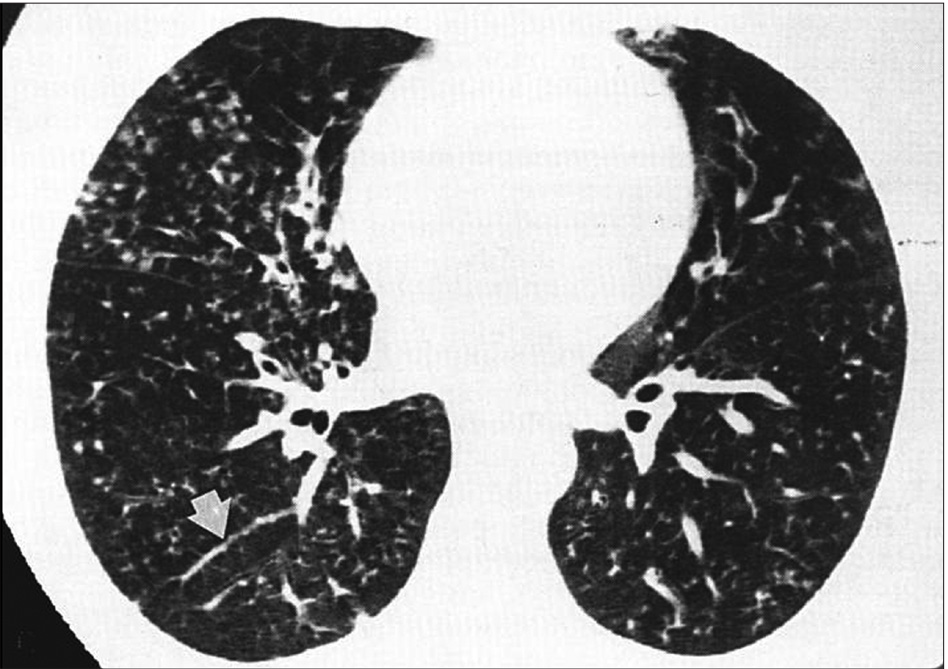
Lymphocytic interstitial pneumonitis in a human immunodeficiency virus patient. High-resolution computed tomography shows smooth and nodular thickening of bronchovascular bundles, centrilobular and subpleural nodularity, ground-glass opacification and interlobular septal thickening. Cysts and mediastinal adenopathy may also be seen (not shown here)
